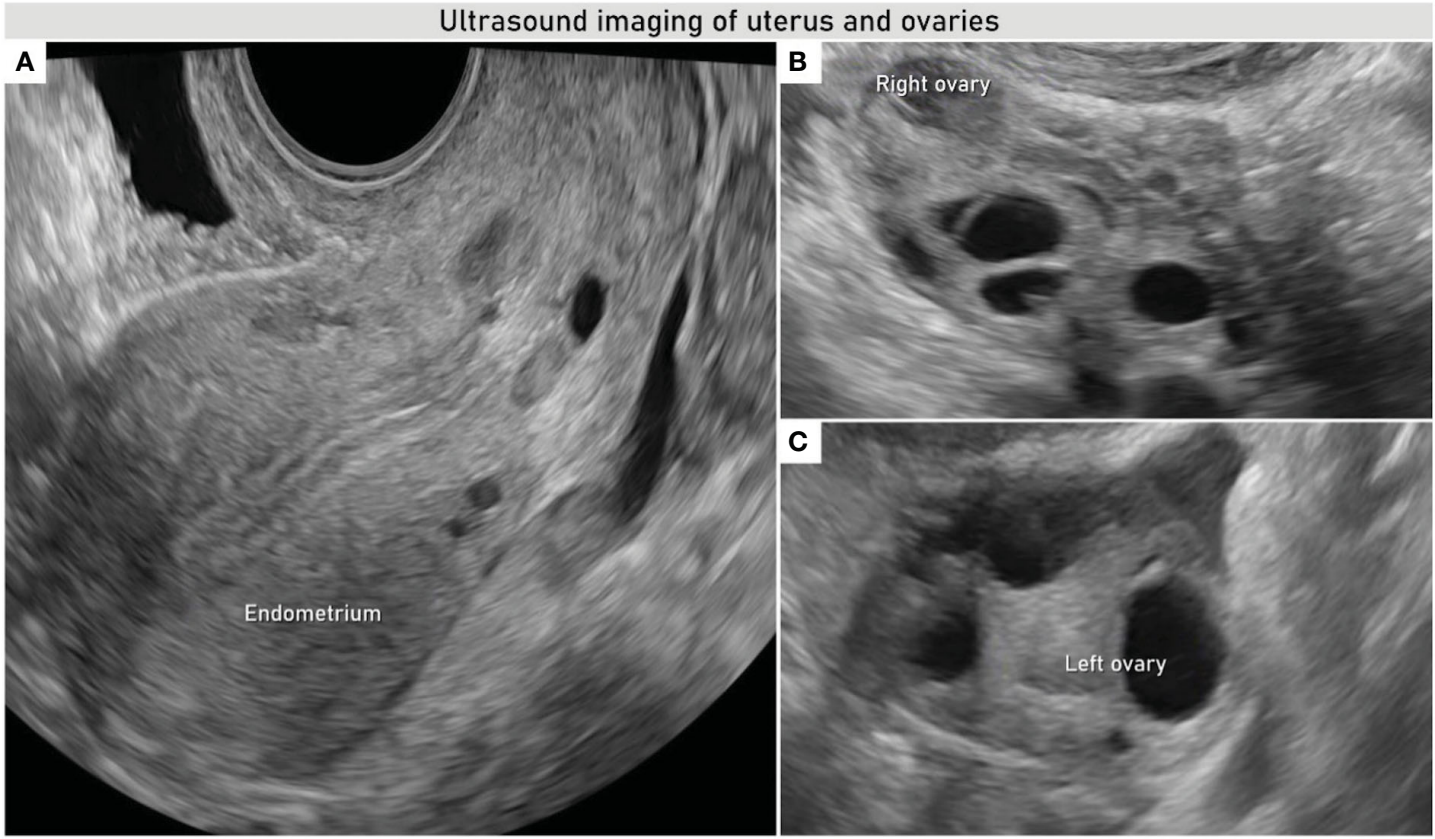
Transvaginal ultrasound evaluation of uterus and adnexa. Uniform endometrium (A) and right and left ovaries of normal appearance (B, C).
